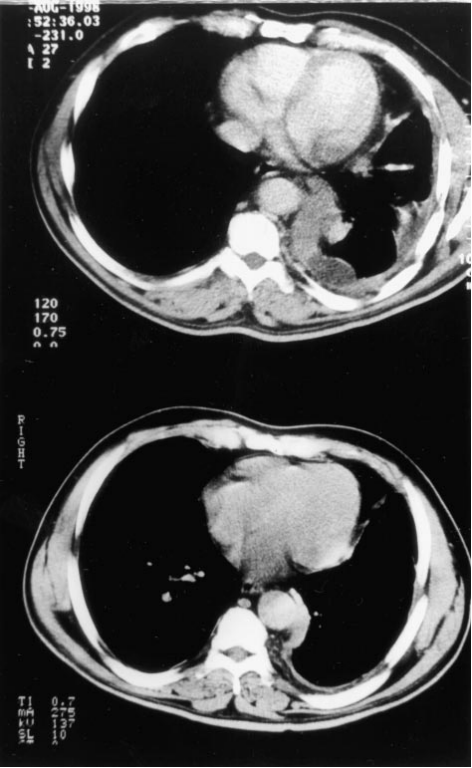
Pre- and post-treatment CT scan of responding patient.
